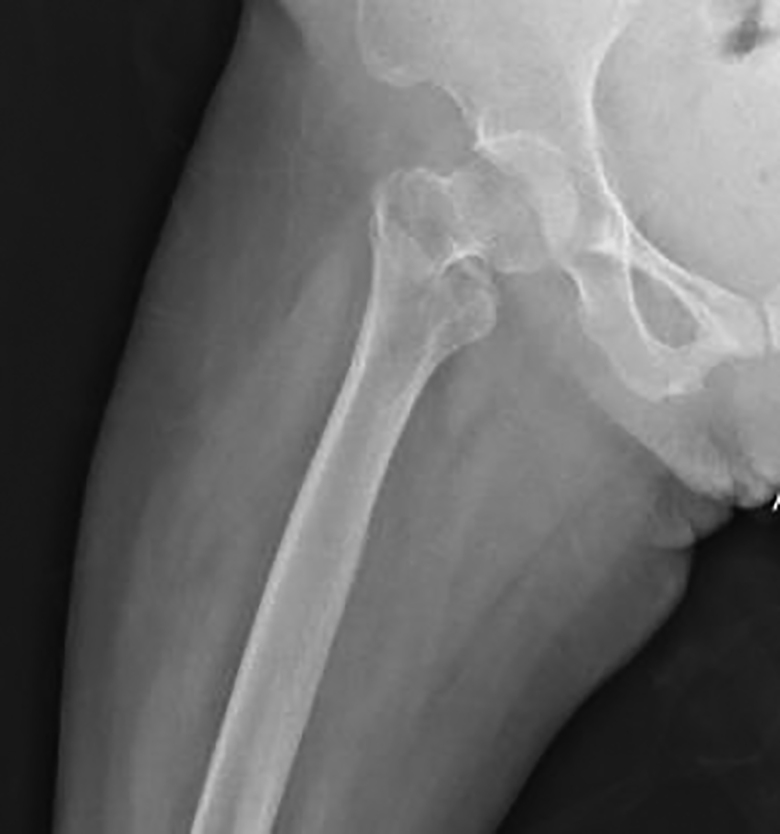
Coronal CT reconstruction of the right intertrochanteric fracture. CT = computed tomography.
